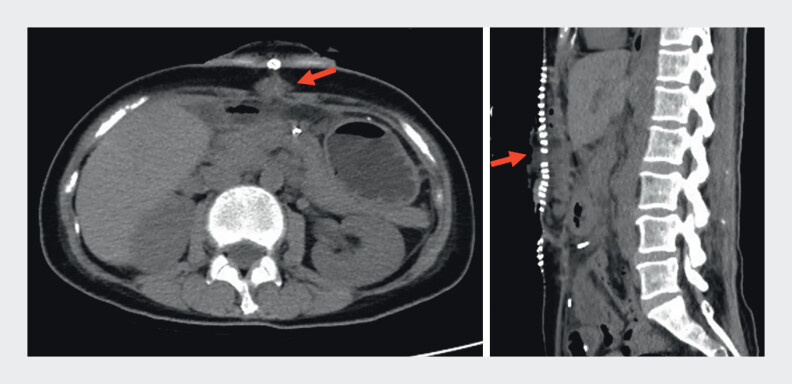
Computed tomography scan showing fluid collection at the surgical site with a tract extending to the abdominal wall (red arrow).
